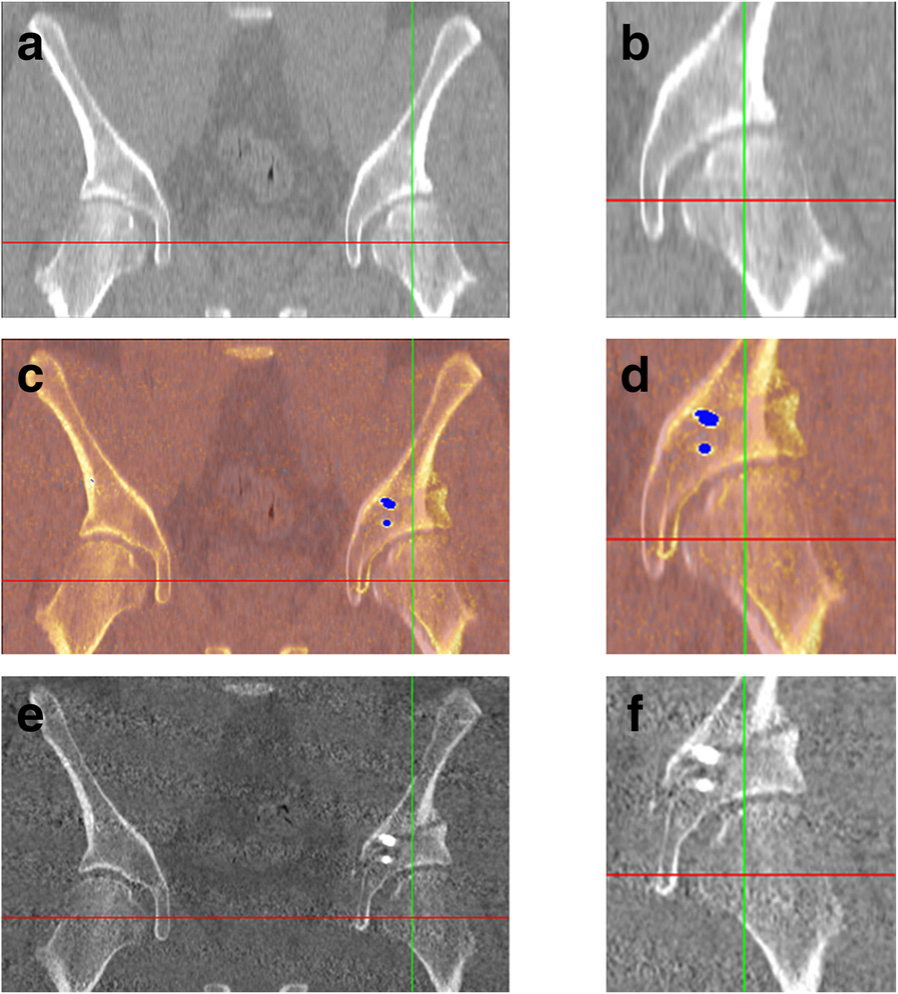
Example of the pre- and postoperative axial CT slices. The CE angle measured automatically from X-ray projections changed from 21° preoperatively to 29° postoperatively. a Preoperative CT slice and b a zoomed in region about the acetabulum. c Preoperative CT slice with a postoperative overlay and d a zoomed in region about the acetabulum. In these displays, the postoperative was highlighted with a color-based filter to distinguish it from preoperative; the *blue dots* are coloring effects due to the intensity of the fixation screws. e Postoperative CT slice and f a zoomed in region about the acetabulum
